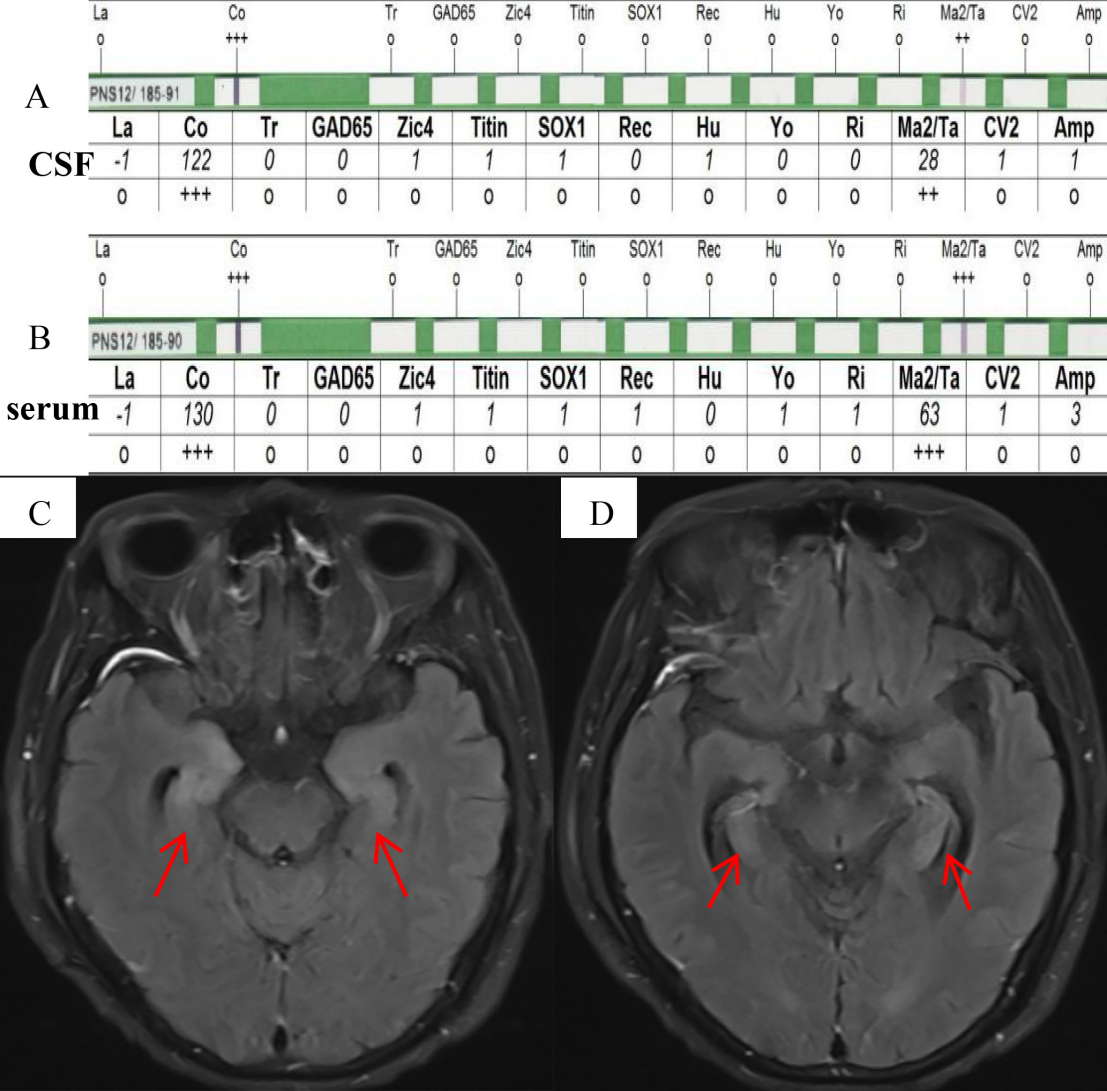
(A, B) Western blot analysis showed a positive anti-Ma2 antibody, corresponding to a high-intensity band in CSF (C) and a higher-intensity band in serum (D). (C, D) FLAIR magnetic resonance imaging revealed abnormal regions characterized by elevated signal intensity in the bilateral mesial temporal lobes, amygdala, and hippocampus (red arrows).
